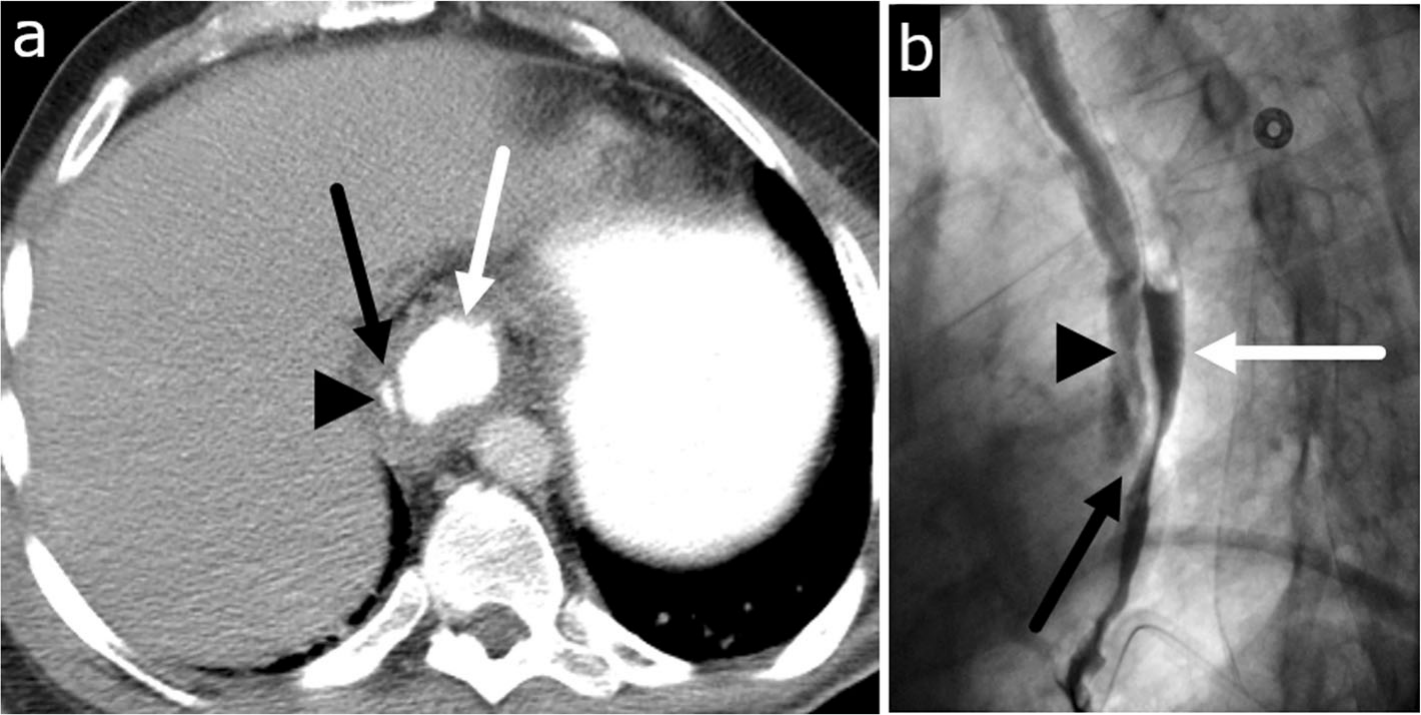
Intramural dissection. Fifty-one-year-old male with chest pain radiating to the back after forceful vomiting. a Axial CT image shows an intramural dissection flap (arrow) between the true (arrowhead) and false lumen (white arrow) in the lower esophagus. b Barium esophagography shows two parallel lumens and an intervening dissecting flap filling defect (black arrow) giving the esophagus the double barrel appearance. True lumen (arrowhead). False lumen (white arrow)
